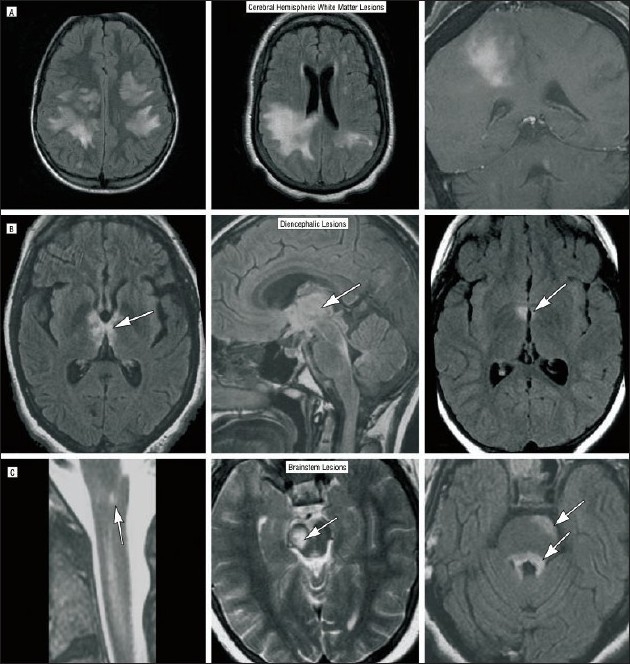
Atypical brain lesions encountered in patients with NMO as seen on MRI. (A, left) Extensive bihemispheric, subcortical, nonenhancing white matter fluid-attenuated inversion recovery (FLAIR) signal abnormality. (A, center) A large confluent FLAIR signal abnormality in the right parietal area that demonstrates diffuse gadolinium enhancement (A, right). (B) FLAIR abnormality in the hypothalamus (B, right, arrow) and the right cerebral peduncle (C, center, arrow). (B, center) FLAIR signal abnormality in the thalamus (arrow), hypothalamus, and optic chiasm, extending into the superior cerebellar peduncle and the floor of the fourth ventricle. (B, left) A confluent nonenhancing signal abnormality extending from the anterosuperior thalamus-hypothalamus (arrow) to the optic tracts behind the chiasm to the superior surface of the mesencephalon extending to the periaqueductal area (right, left) to the superior cerebellar peduncles, and the pontine tegmentum (C, right, arrows). Extension of T2-weighted MRI signal abnormality into the medulla (C, left, arrow) in a patient with an otherwise normal brain MRI. Reprinted with permission from Sean Pittock (Mayo Clinic Rochester, USA) and Arch Neurol. 2006 Mar; 63(3): 390-6(25). Copyright American Medical Association, 2003, American Medical Association, All rights reserved.
